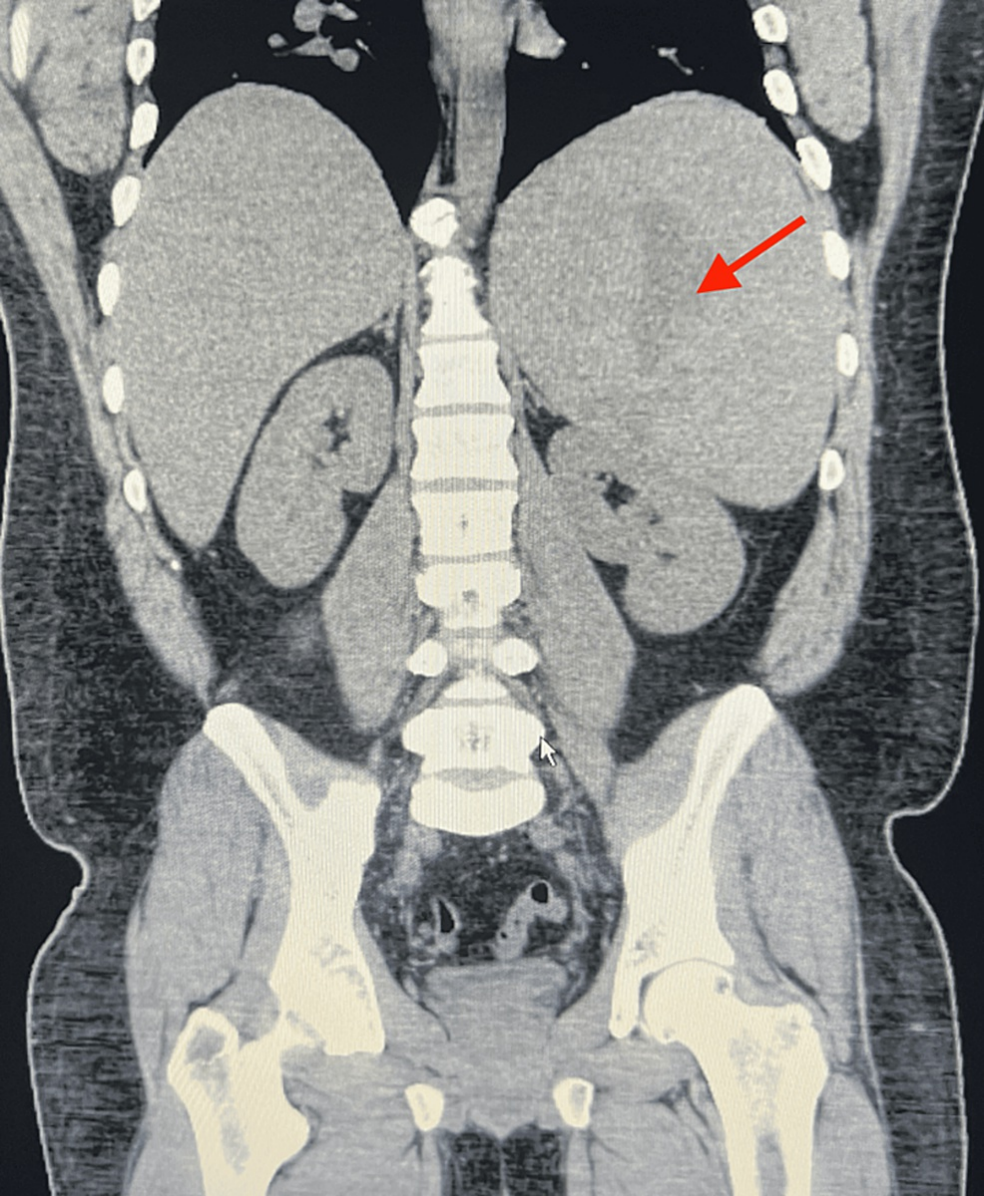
CT of the abdomen and pelvis showing marked splenomegaly
